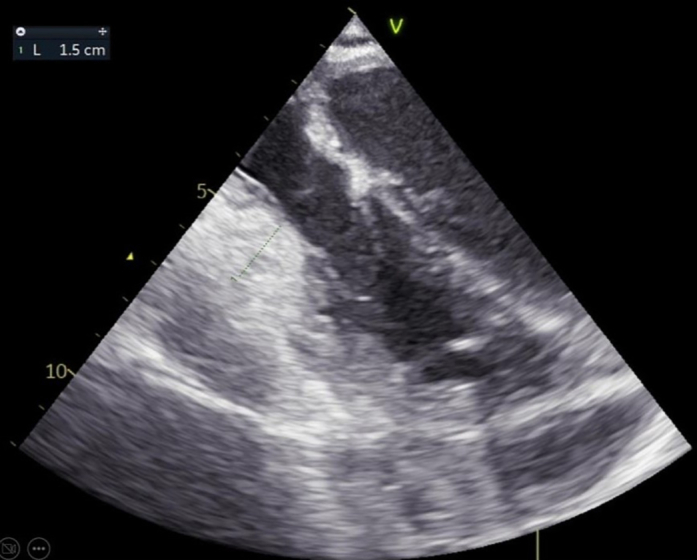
Mediastinal Hematoma Transesophageal echocardiography showed a mediastinal hematoma, which required surgical evacuation.
